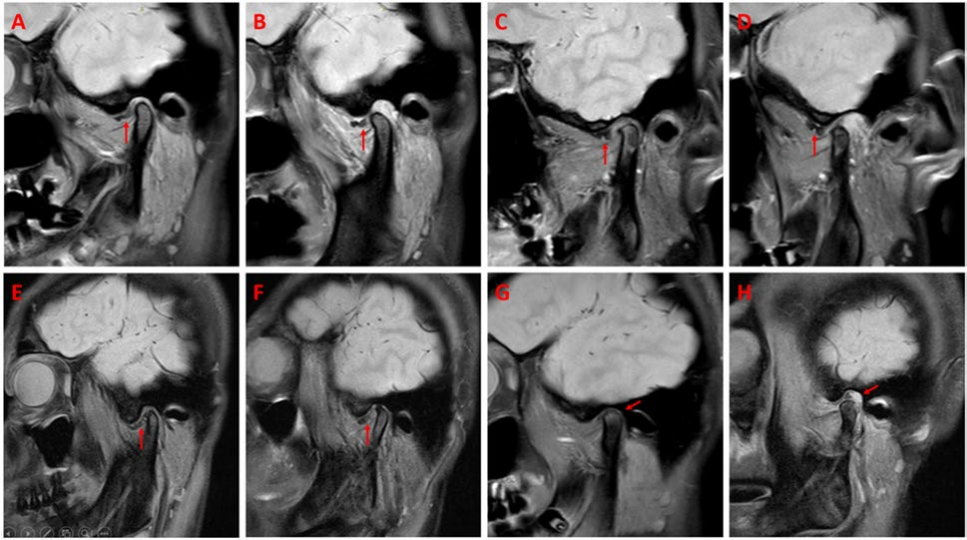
Representative MRI features associated with LMO in TMD patients. A, B Case 1 of ADDwoR. A Closed-mouth MRI showings anterior disc displacement (red arrow); (B) Open-mouth MRI demonstrating persistent nonreduction (red arrow). C, D Case 2 of ADDwoR. C Closed-mouth MRI showing anterior disc displacement (red arrow); (D) Open-mouth MRI demonstrating persistent nonreduction (red arrow). E, F Abnormal disc signal (red arrows). G, H Abnormal joint space. G Narrowed joint space (red arrows); (H) Widened joint space (red arrows).
